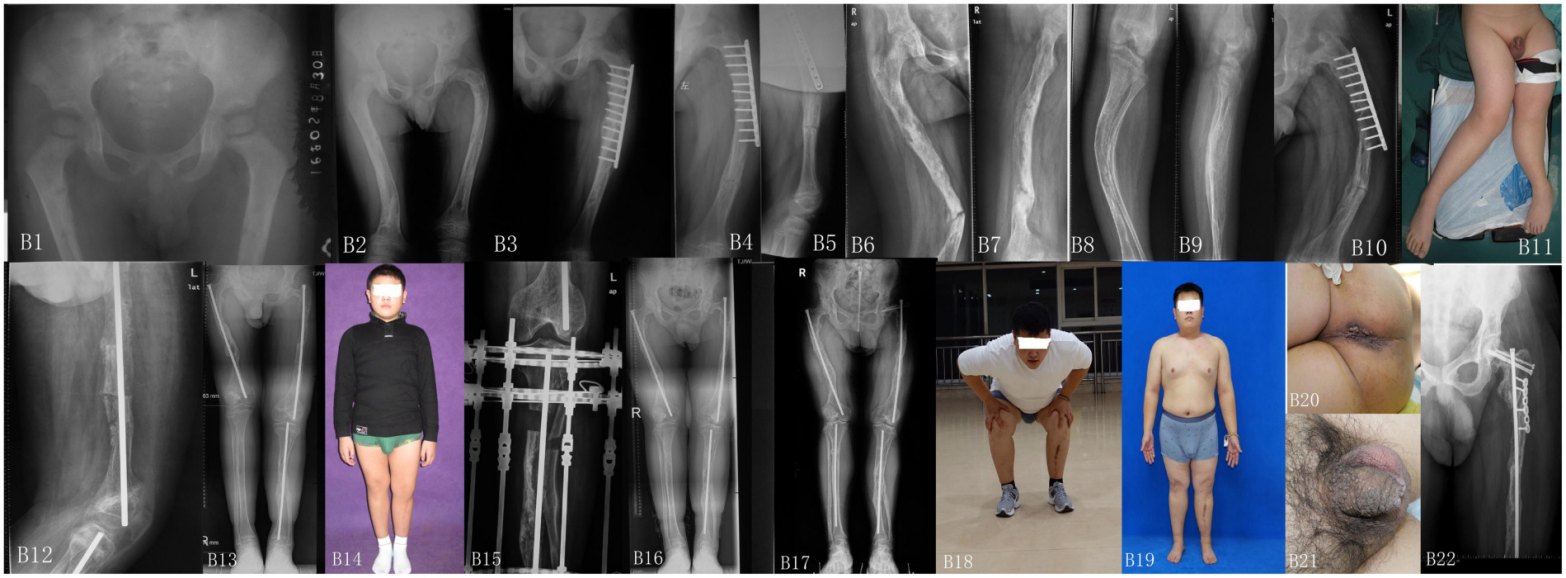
B1. At age 2, bilateral proximal femoral fractures with severe osteoporosis. B2. At age 6, recurrent fractures with malunion and obvious bowing deformities of the bilateral femurs. B3. At age 7, left femoral fracture treated with plate osteosynthesis at an external institution. B4–B5. At age 8 (1-year post-surgery), plate dislodgement with distal femoral fracture. B6. At age 12, right femoral fracture with distal bowing deformity. B7. Left femoral osteoporosis and bowing deformity. B8–B9. X-ray of left tibia. B10. At age 12, complete dislodgement of the left femoral plate withrecurrent deformity. B11. At age 12, severe bilateral lower limb bowing. B12. left femoral multilevel osteotomy with P-W intramedullary nailing. Postoperative radiograph showed significant improvement in femoral bowing deformity and restoration of satisfactory mechanical alignment. B13. At age 15, radiographs confirmed maintained correction of bilateral femoral and left tibial deformities with stable implants. All osteotomy sites achieved bony union and bone density increased. B14. At age 15, asymmetricallimb length and corrected alignment during weight-bearing were noted. B15. At age 16, the patient underwent left tibia-fibula osteotomy with external fixation for correcting limb length discrepancy. The lengthening outcome was tisfactory. B16. At age 17, the osteoto my site showed good healing. B17. At age 22, the patient sustained right tibial fractures and underwent open reducti on and internal fixation (ORIF), with satisfactory postoperative healing. B18–B19. At age 25, hip joint mobility was preserved, squatting was slightly limited, the lower limbs were nearly equal in length with no significant deformit ies, and surgical healing was good. B20–B21. Numerous epdermal nevi were noted around the anus and penoscrotal regio n during surgery. B22. At age 26, the patient underwent left valgus-producing proximal femoral osteotomy with plate.
